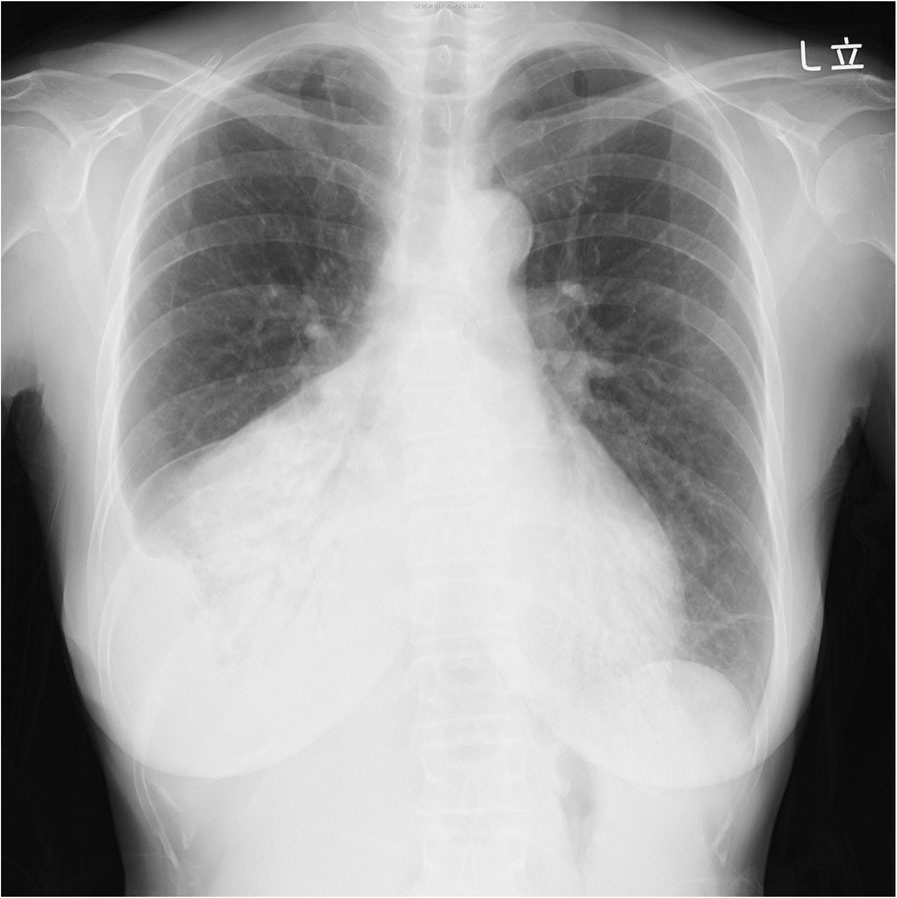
Chest x-ray image on admission
